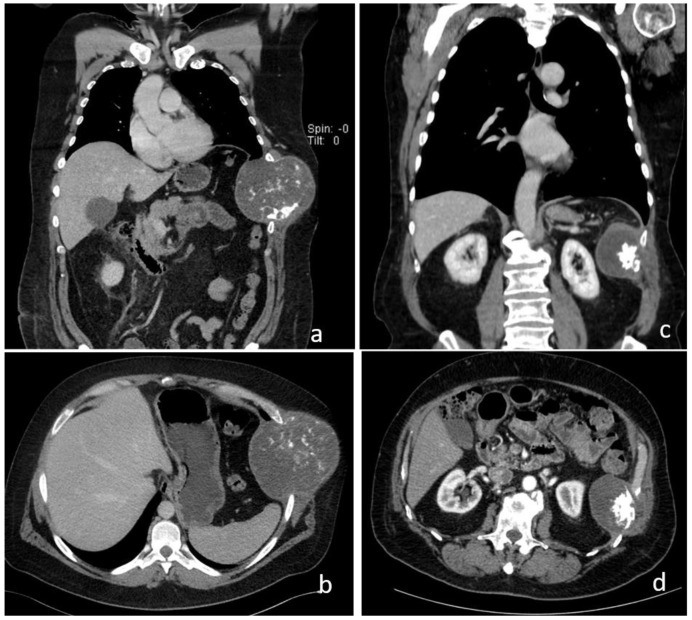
Computed tomography findings of two patients with primary chondrosarcoma of the chest wall in coronal (a,c) and axial reconstruction (b,d). Tumor involving the 7th and 8th ribs (a,b). Tumor involving the 9th and 10th ribs (c,d).
